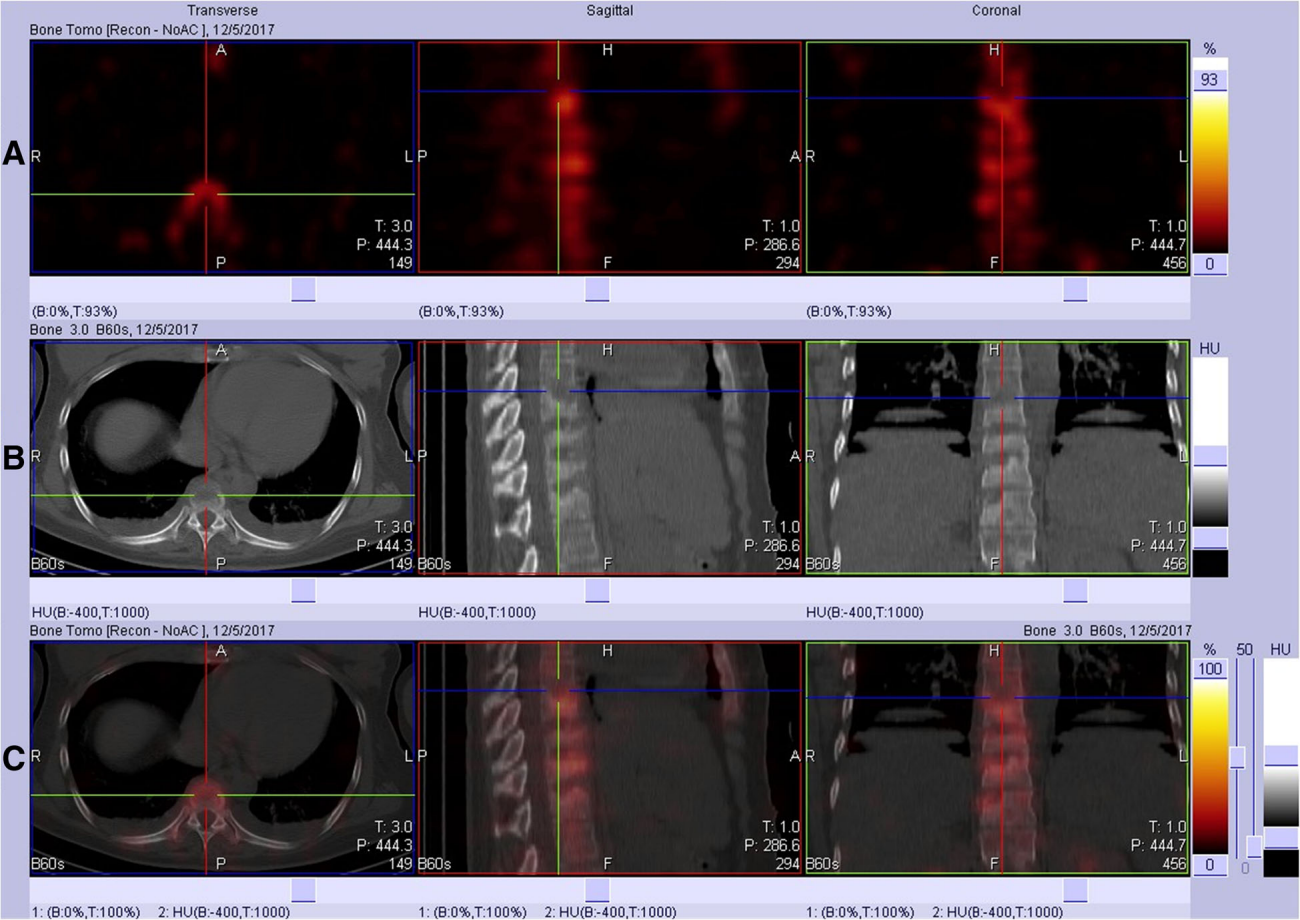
SPECT/CT imaging of thoracic and lumbar revealed marginal erosions and superior endplate compression deformity in the T8–11 vertebral bodies. a: SPECT imaging; b: CT imaging; c: Fusion imaging
